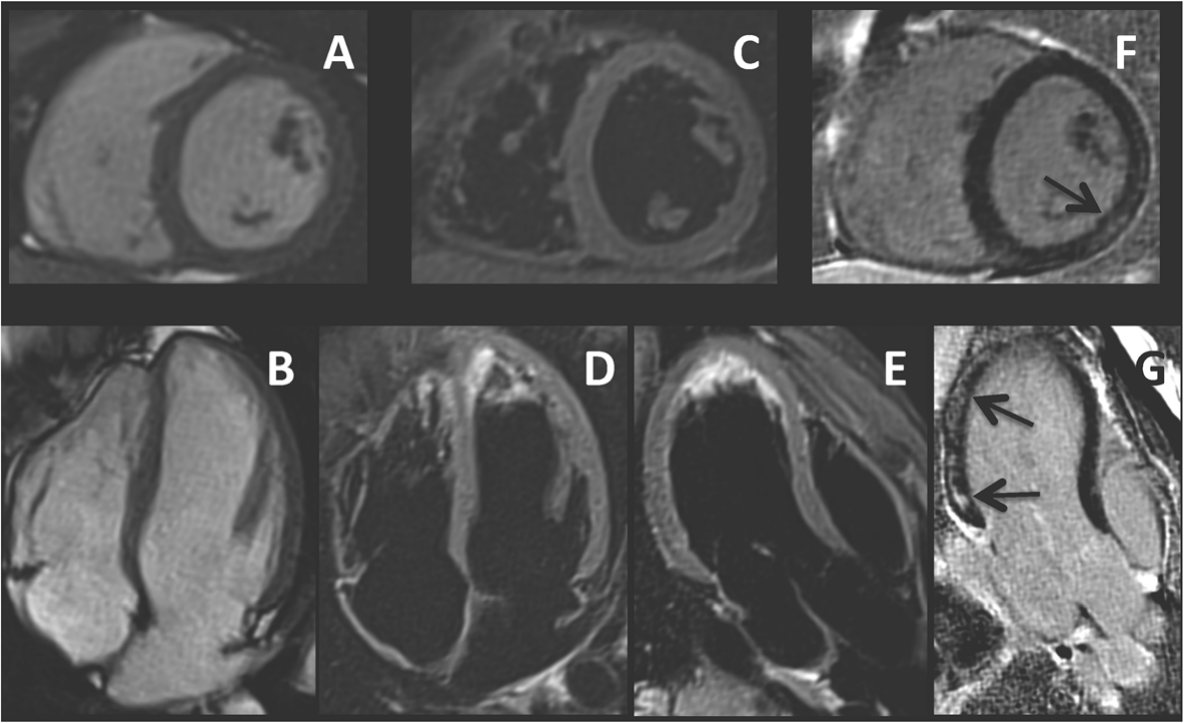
Cine and late gadolinium enhancement (LGE) CMR images of the patient at baseline. Panel A and B: cine (steady-state free precession sequence) CMR of short-axis (A) and 4-chamber (B) view showing normal left ventricular wall thickness. Panel C, D and E: T2-weighted short-TI inversion-recovery fast spin-echo image of short-axis (C), 4-chamber (D) and 3-chamber (E) view showing the absence of myocardial oedema. Panel F and G: contrast-enhanced inversion recovery gradient echo image of short-axis (F) and 3-chamber (G) view showing LGE involving the inferolateral wall with intramyocardial distribution (black arrow).
